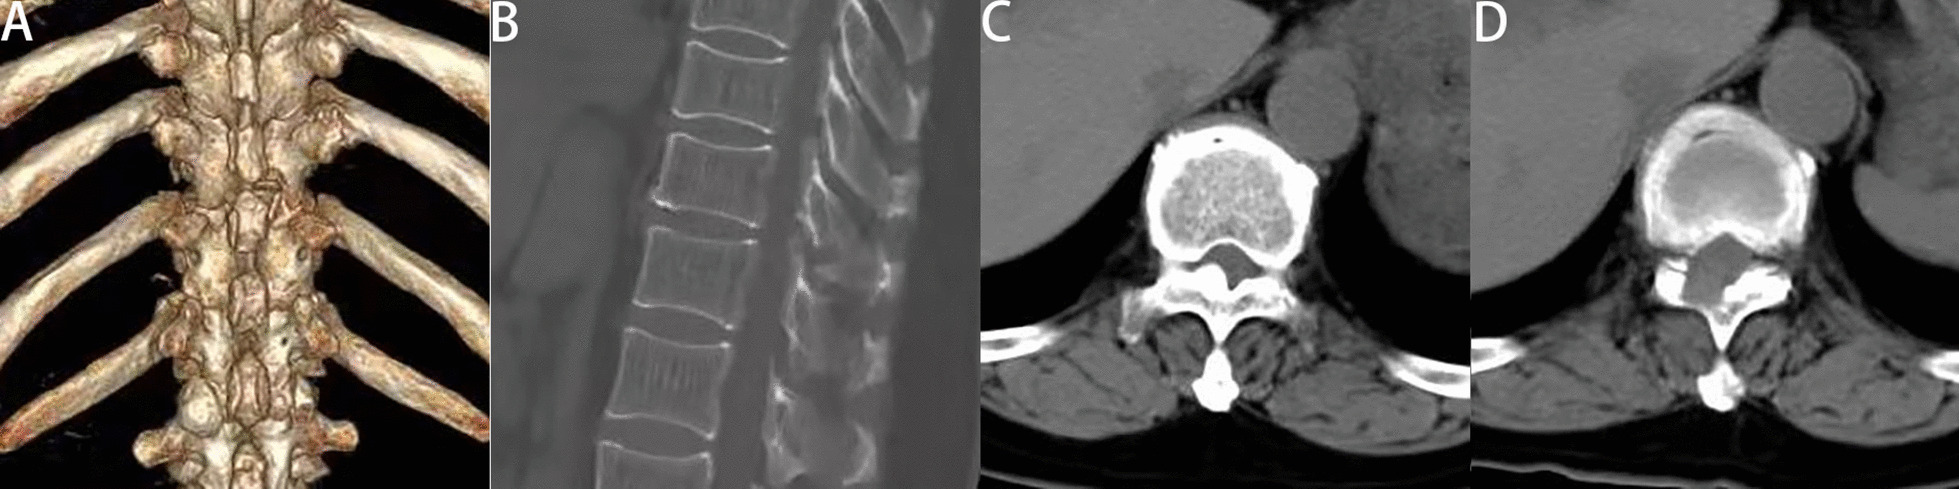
A Postoperative 3D reconstruction showed the location of the working channel. Compared with the preoperative CT (Fig. 2A), the postoperative CT (sagittal) (B) revealed that the OLF was completely removed. Computed tomography of the thoracic spinal cord showed that adequate decompression was performed. A Preoperative CT (axial). B Postoperative CT (axial)
